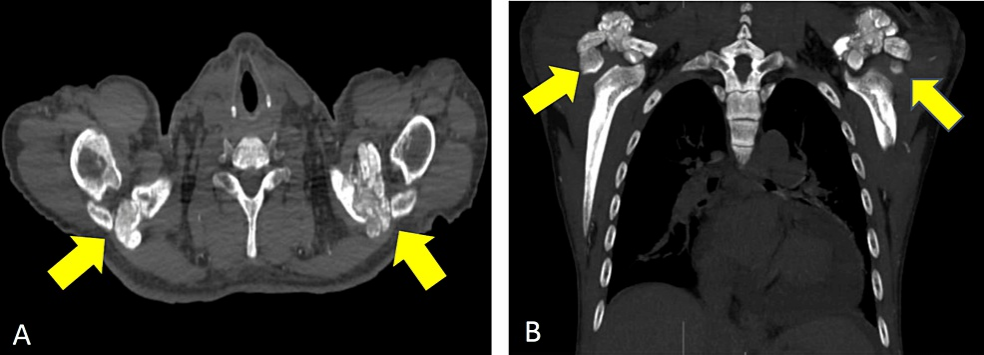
Plain CT of the chest (A) Axial and (B) coronal views showing amorphous, multilobulated masses in both acromioclavicular joints (arrows). The masses measure 4.5x4.1x2.8 cm (CCWAP) in the right and 3.5x4.8x4.3 cm (CCWAP) in the left. CT: computed tomography
